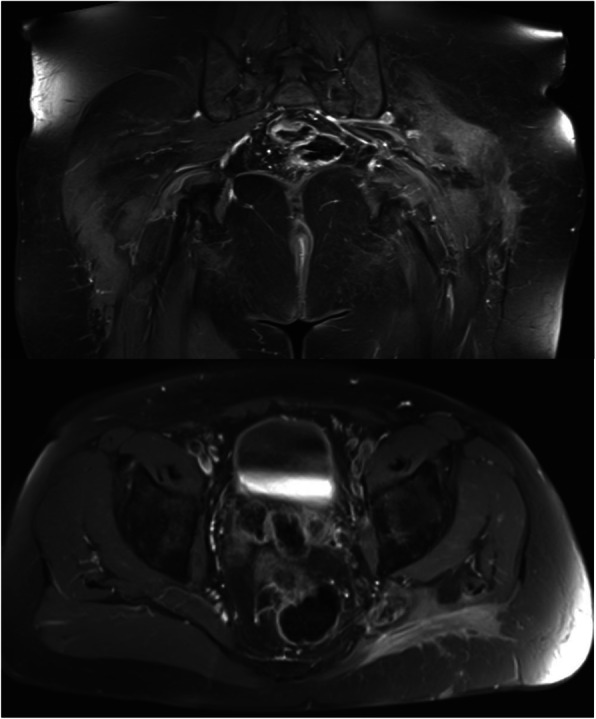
MRI follow up 12 months after surgical resection
